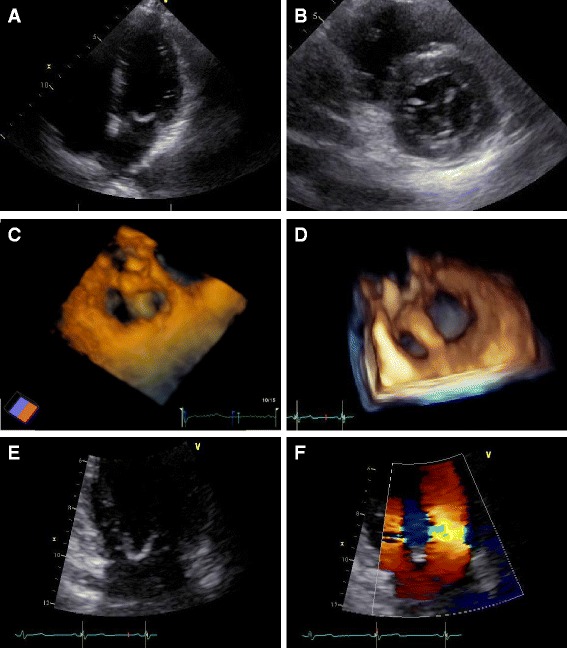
Trans-thoracic echocardiography demonstrating the DOMV in a modified 4-chamber view (a), a 2-dimensional short-axis (b) and two 3-dimensional images (c, d) demonstrating the two mitral orifices, and the fibrous bridge separating orifices. Zoomed images (e, f) demonstrate the separate inflow jets through the orifices.
